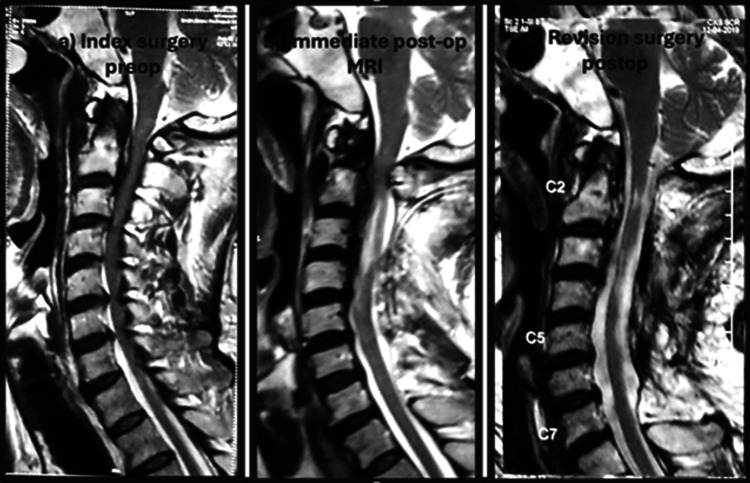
Images from the redo cervical laminectomy case. (A) Preoperative MRI showing stenosis at C2-C6 levels. (B) Immediate postoperative MRI after the index surgery demonstrating inadequate decompression (arrow). (C) Post-revision MRI following C1 arch excision showing adequate decompression.
